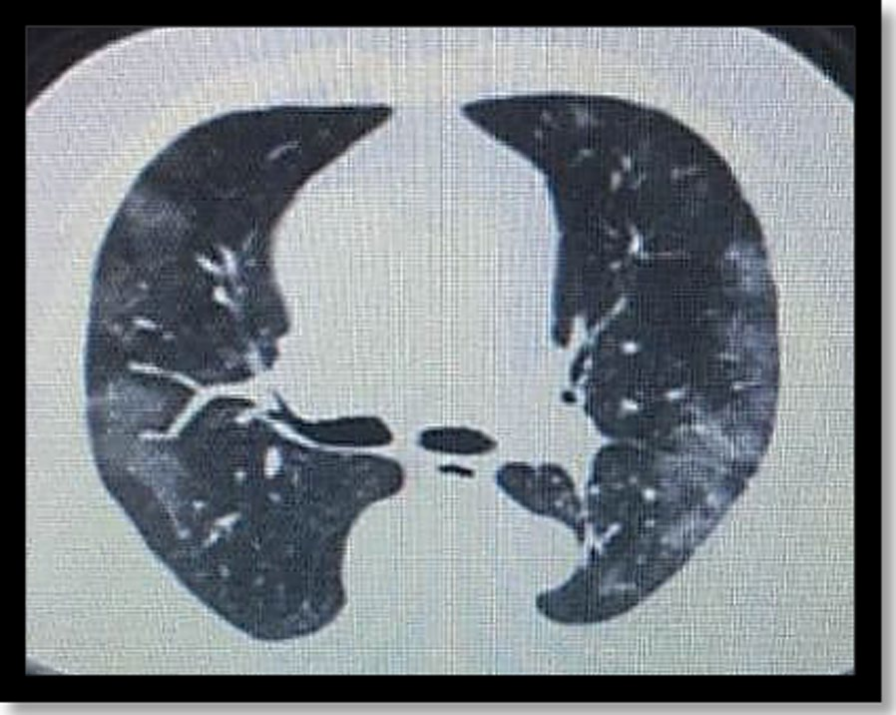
Axial nonenhanced chest CT of the patient showing bilateral patches of ground-glass opacities in a peripheral and sub-pleural distribution, diagnosing COVID-19 pneumonia
